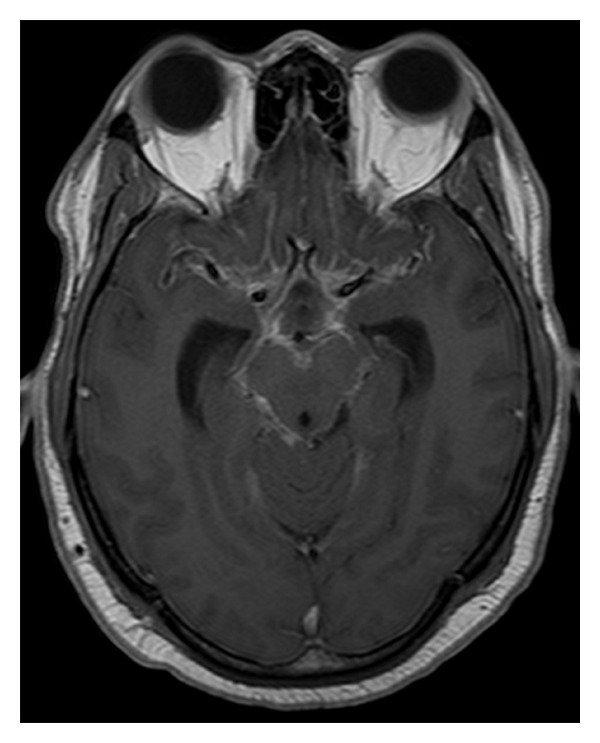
Cerebral T1-weighted MRI showing diffuse leptomeningeal carcinomatosis.
